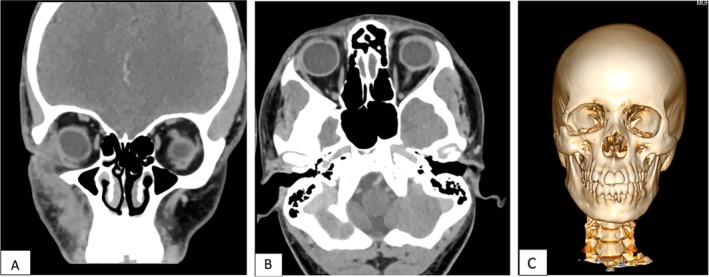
(A, B) 1‐year post‐operative CT scan, coronal and axial views showing no signs of recurrence. (C) CT scan 3D reconstructed view showing the lateral orbital rim defect that was unrepaired.
